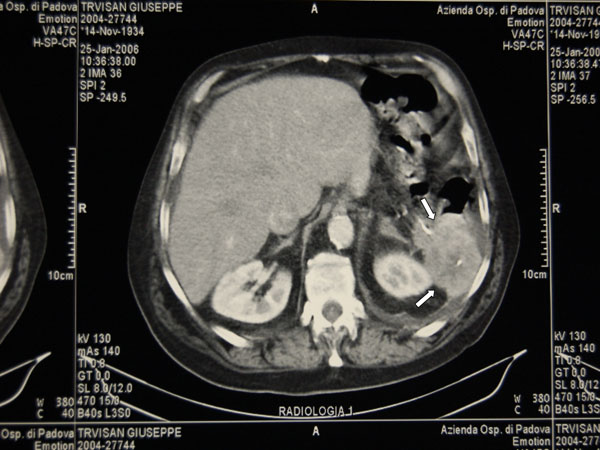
CT scan demonstrating a bulk in splenic area of about 8 cm in diameter and infiltrating pancreatic tail with adhesions to left diaphragm, left colic flexure and left kidney fascia
